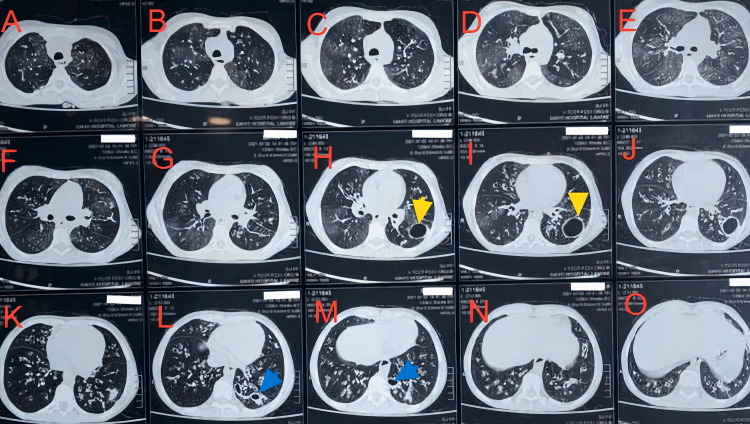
HRCT chest, axial sections Sections A to H show diffuse mosaic attenuation, the yellow arrows in sections H and I point to a cavity formed from repeated infections, and the blue arrows in sections L and M show signet ring sign, which depict bronchiectasis HRCT: high-resolution computed tomography
